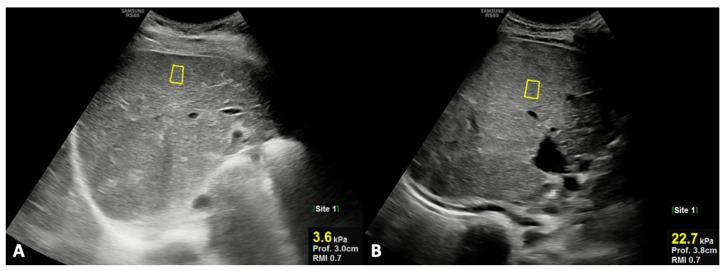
Ultrasound oblique scans under the right rib, showing shear-wave elastography measurements using ROI analysis in a patient of group 1 ((A) #5; Table 1—liver stiffness = 3.6 kPa) and in a patient of group 2 ((B) #11; Table 2—liver stiffness = 32.5 kPa).
